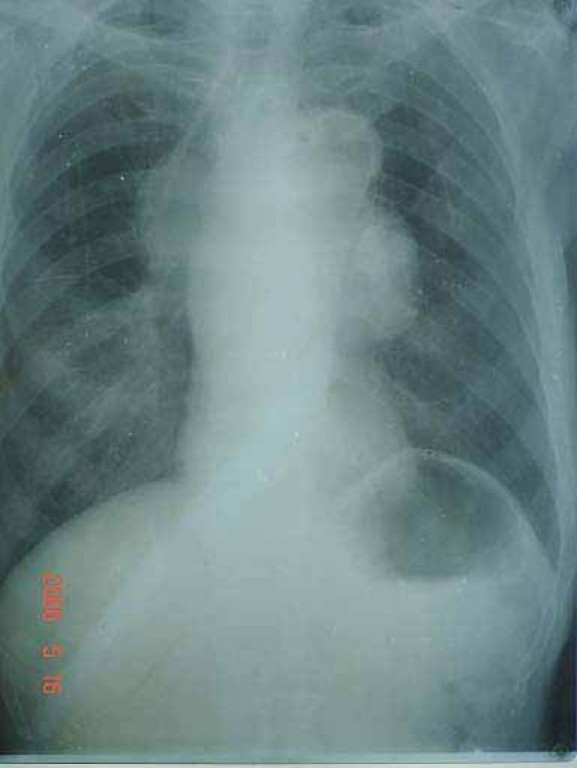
Chest X-Ray PA view showing mediastinal mass
